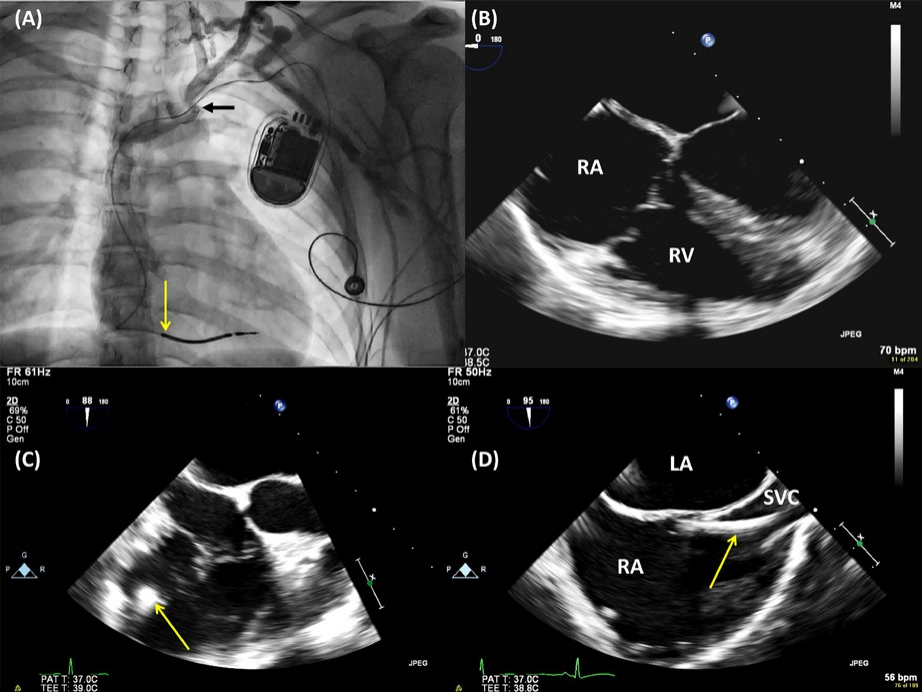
A, Left arm phlebography. The subclavian vein stops abruptly (dark arrow), with collateral filling visible above; the right ventricular lead is indicated with an yellow arrow. B, In 2D transesophageal 4‐chamber view, the lead was difficult to image being situated very posteriorly; C, in short axis of the tricuspid valve, the lead becomes visible; D, bicaval view, demonstrating the relation between the lead and the superior vena cava
